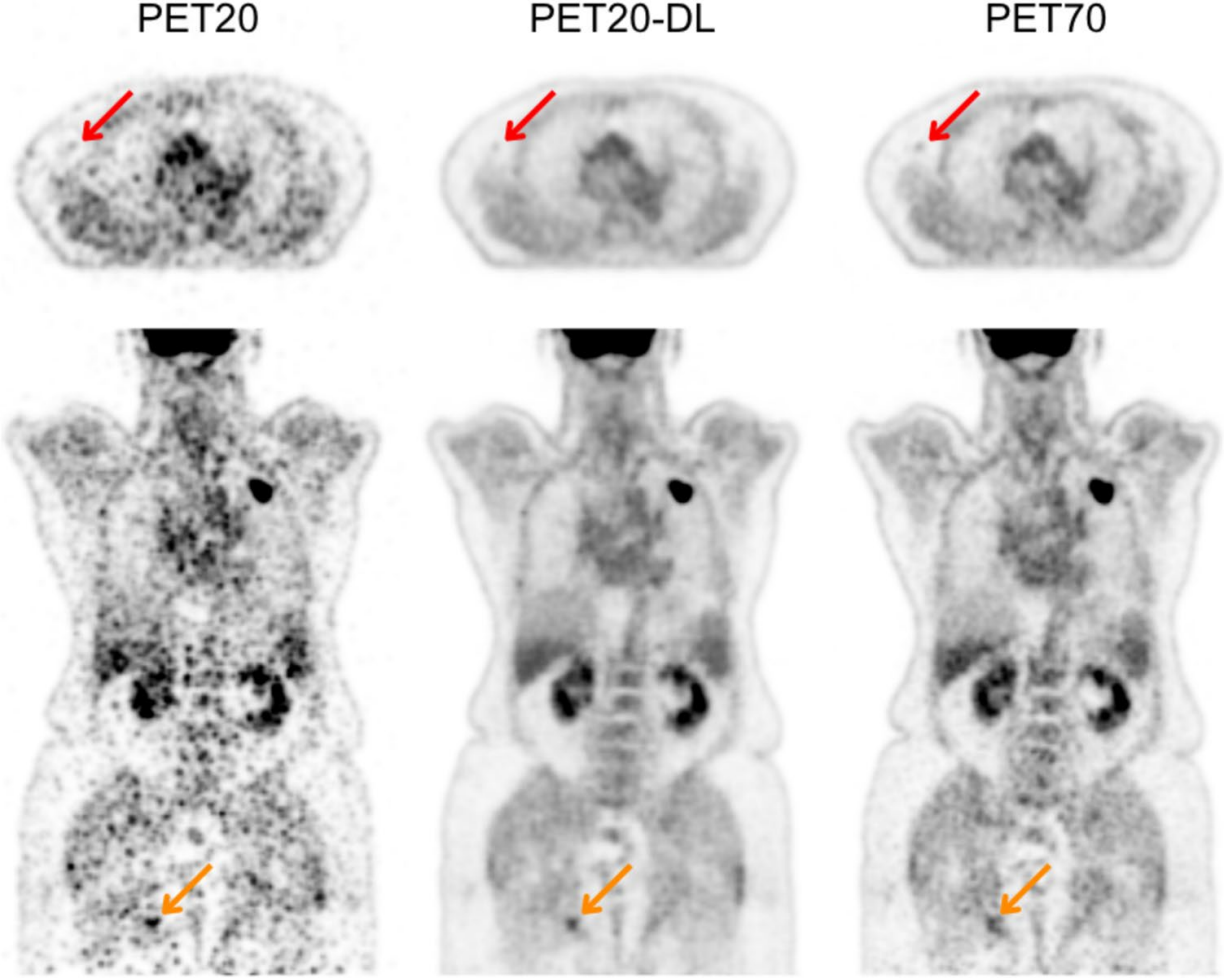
[^18^F]FDG PET acquisition of a patient from the test set, for which a classification of 1 (extremely lower) in visual image quality relative to the standard of care was given by 2/3 readers to the 20 s/AFOV acquisition (PET20). A classification of 4 (higher image quality) was given by 1/3 readers to PET20 + DL-based denoising (with the remaining giving a classification of 3, meaning similar image quality to standard of care). A classification of 3 was given by 3/3 readers to the 70-s/AFOV reference acquisition (PET70). Red arrows refer to a false negative—a lesion missed in PET20-DL but detected in PET70. Orange arrows refer to a false positive—a “lesion” detected in PET20-DL but “missed” in PET70
